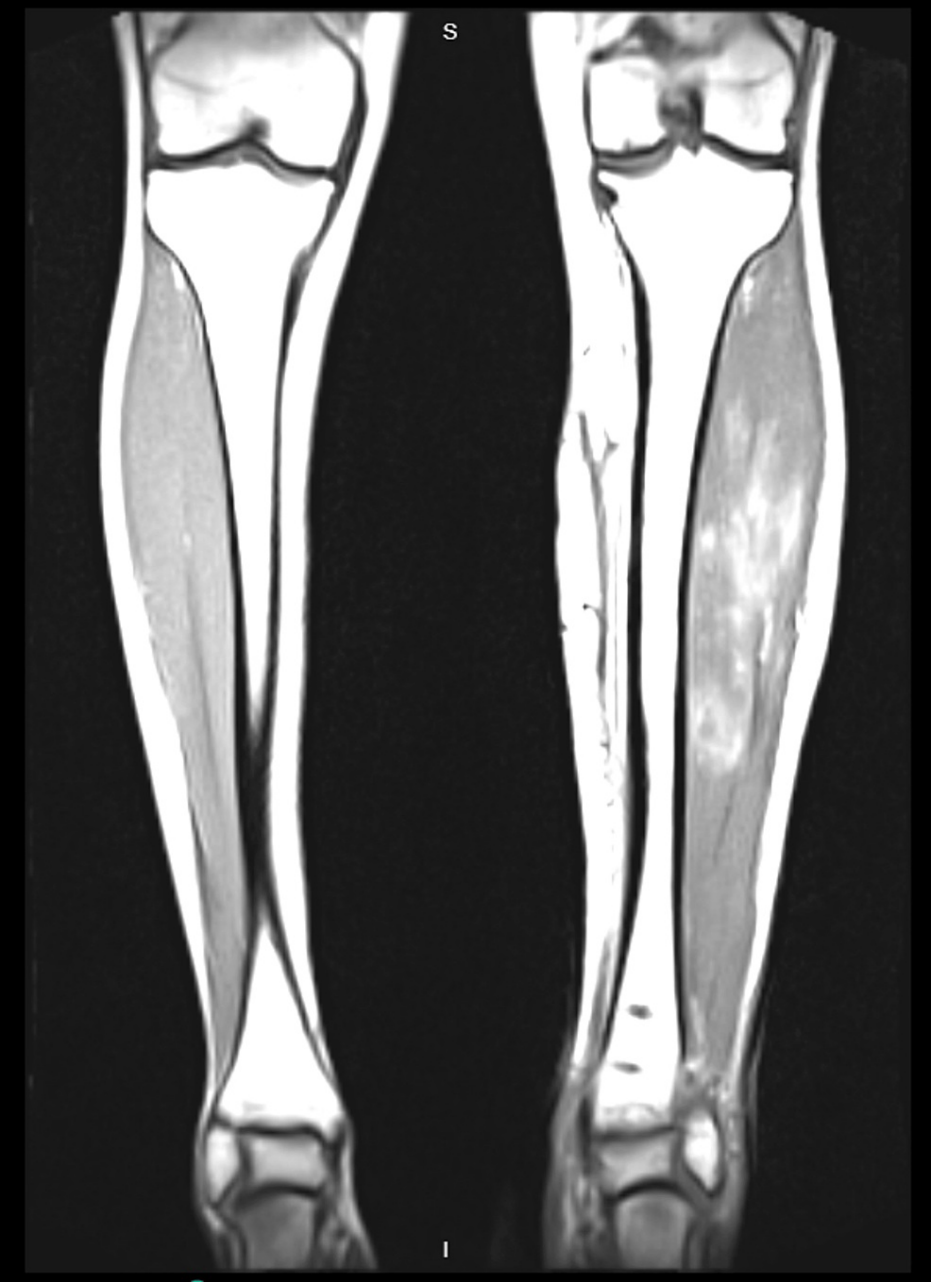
Coronal MRI of left and right legs on day eight post-index procedure. Compartmental oedema of the anterior and deep posterior compartments
